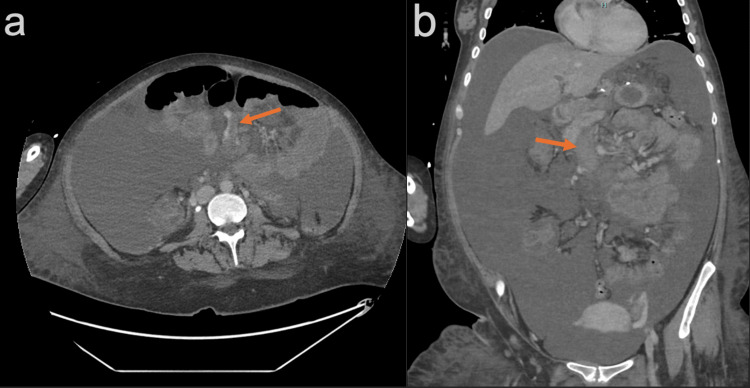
Axial (a) and coronal (b) contrast-enhanced CT of the abdomen demonstrating occlusion of the superior mesenteric vein (arrows).
